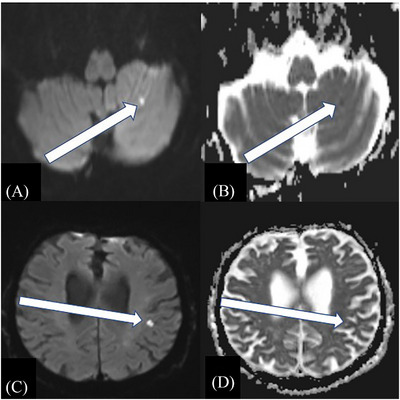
MRI imaging showing an area of hyperintensity on DWI imaging (A) with correlate on ADC imaging (B) consistent with an area of acute ischemic in the left cerebellum. MRI imaging showing an area of hyperintensity on DWI imaging (C) with correlate on ADC imaging (D) consistent with a cortical region of infarct in the left parietal lobe. ADC, apparent diffusion coefficient; DWI, diffusion weighted imaging; MRI, magnetic resonance imaging.
